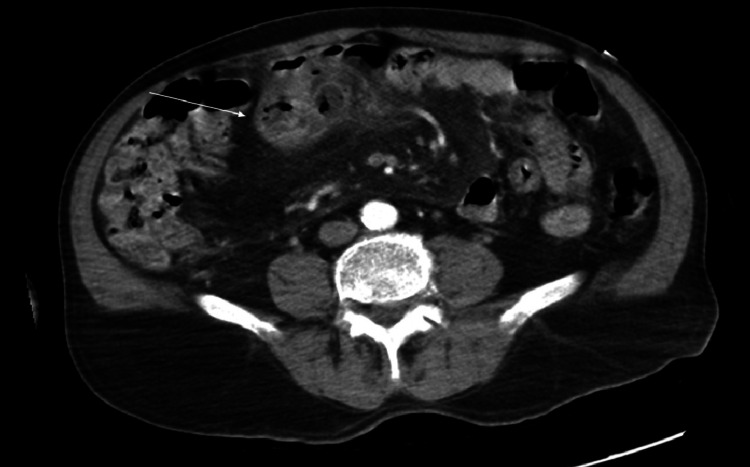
Computed tomography (CT) demonstrating acute jejunal diverticulitis (white arrow).
